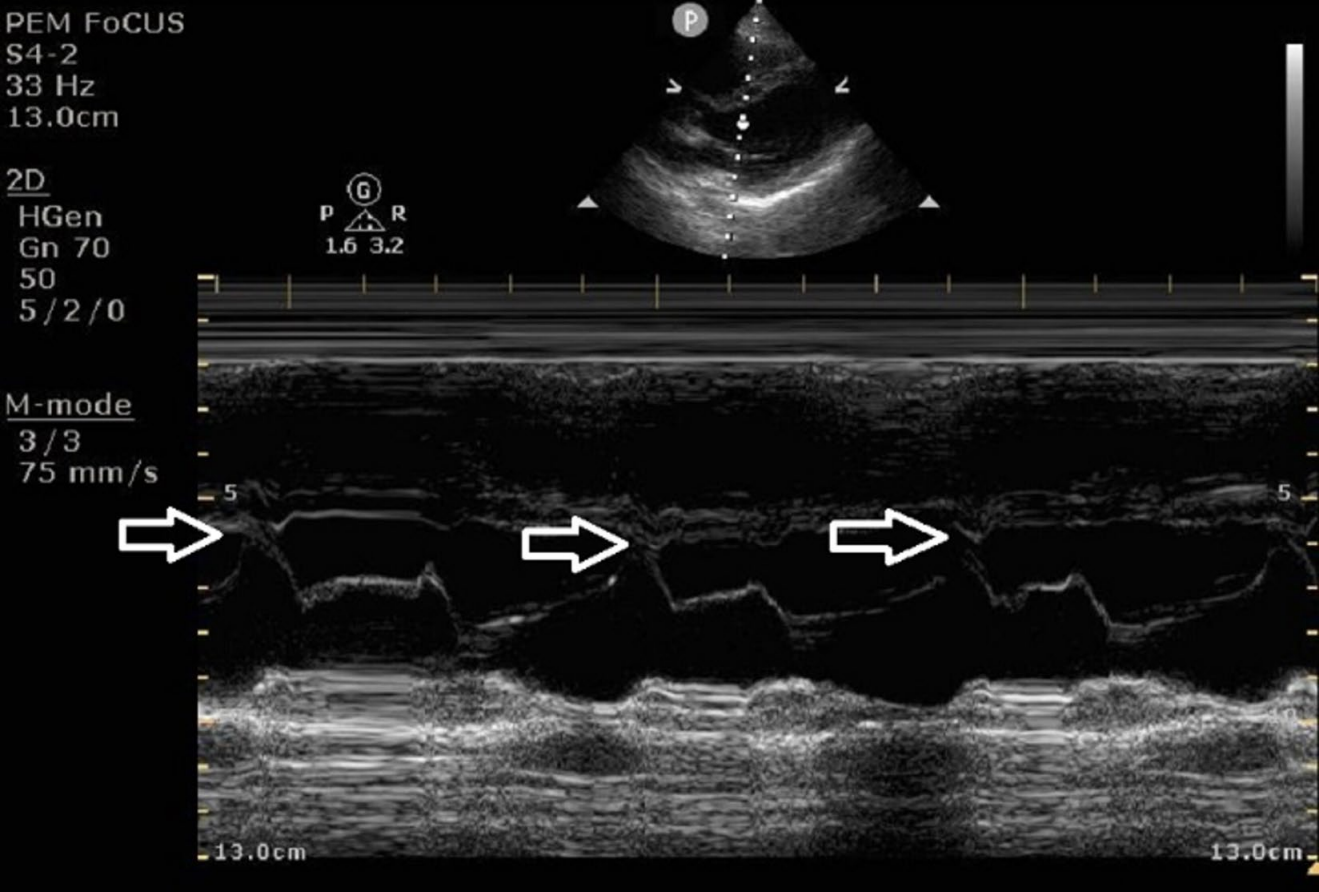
EPSS measurement obtained in the parasternal long axis view. Movement of the anterior leaflet of the mitral valve is seen to contact the septum (arrows) for a measurement of 0 mm
